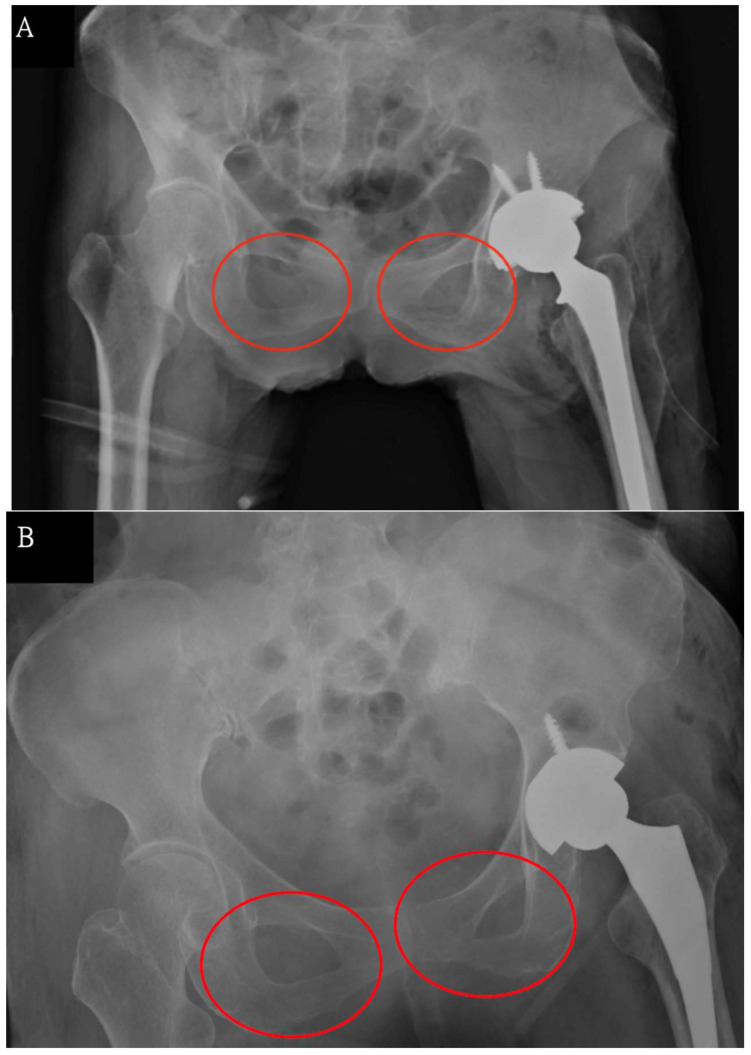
(A,B). Plain radiographs showing pelvic rotation (uneven obturator foramens, marked in red circles).
